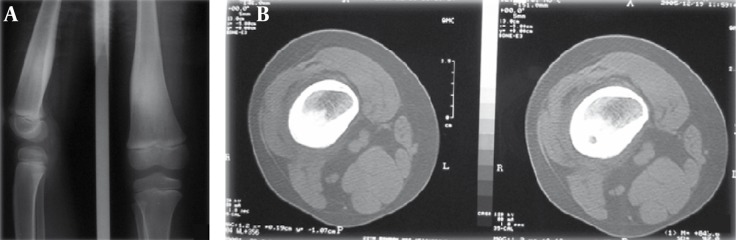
A 17-Year-Old Man Presenting With Thigh Pain. A, Plain radiographs show endosteal thickening at the distal of the femur. No definite radiolucencies are present. B, CT scan reveals the endosteal thickening and demonstrates radiolucencies in the cortex.
